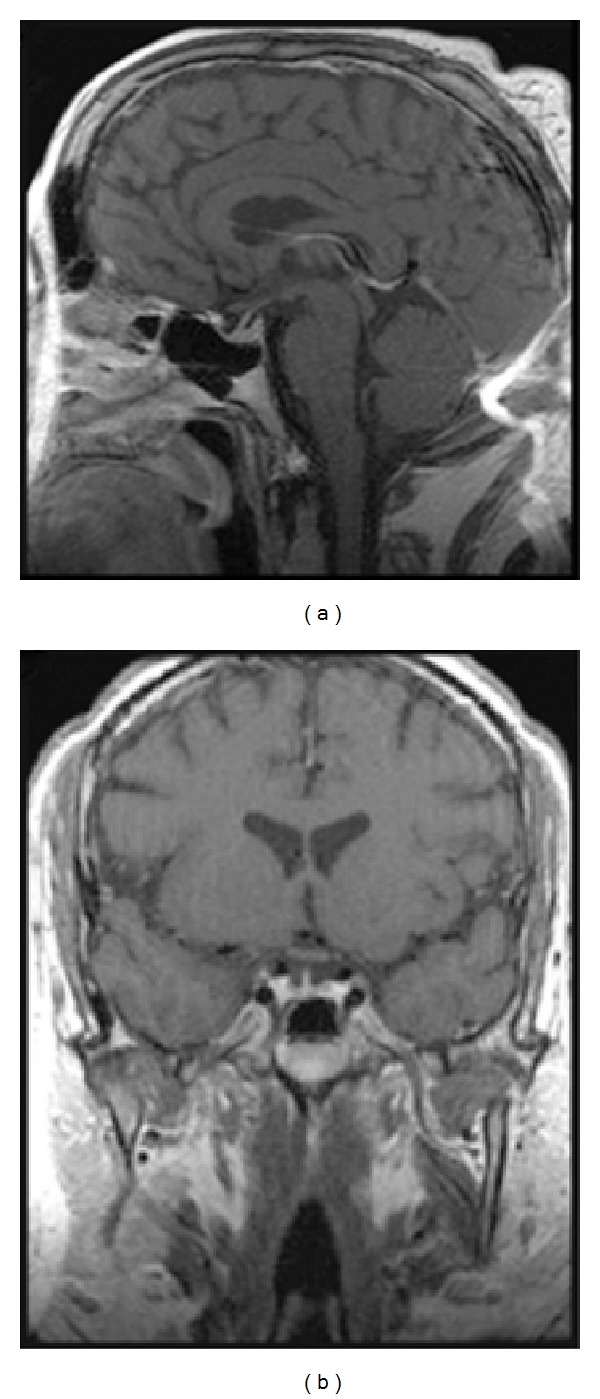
Postoperative MRI at 2 years follow-up.
